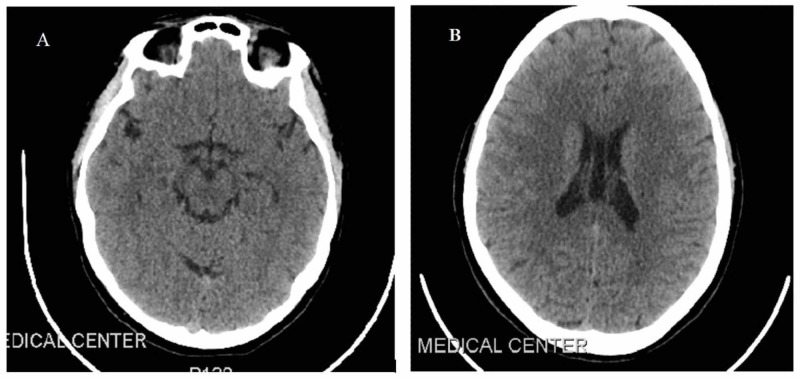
A, B: Non-contrast axial computed tomography brain on admission with no abnormalities
